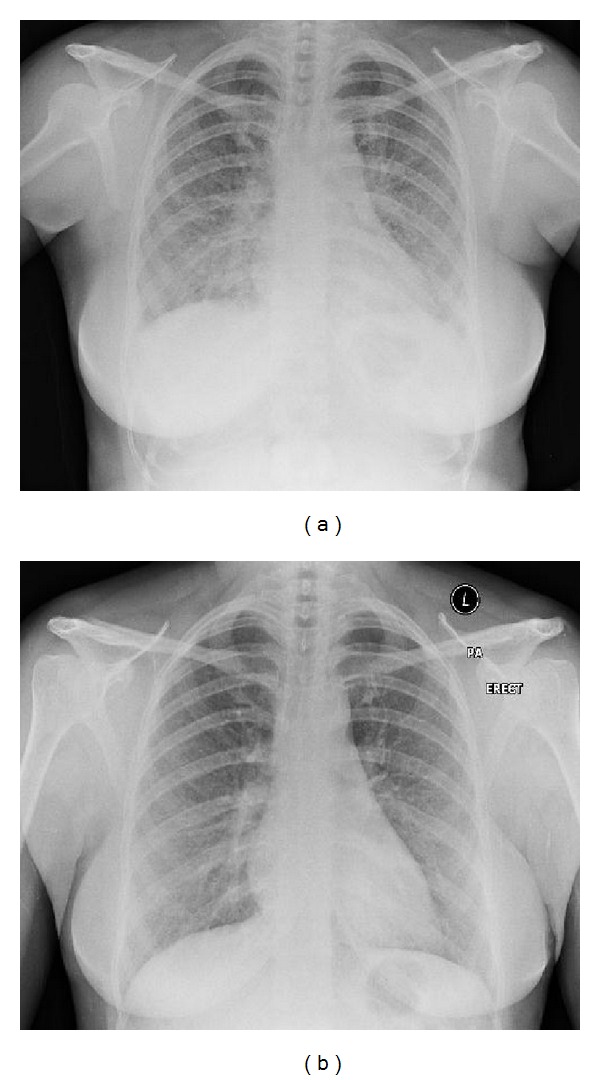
Chest X-ray pre-and postdiscontinuation of anti TNF-*α* therapy and commencement of oral prednisolone. (a) Chest X-ray with widespread reticulonodular shadowing with prominence of the of right hilum suggestive of lymphadenopathy pre-anti-TNF-*α* therapy. (b) Chest X-ray showing improvement in the extent of reticulonodular shadowing post-discontinuation of anti-TNF-*α* therapy and the commencement of oral prednisolone.
